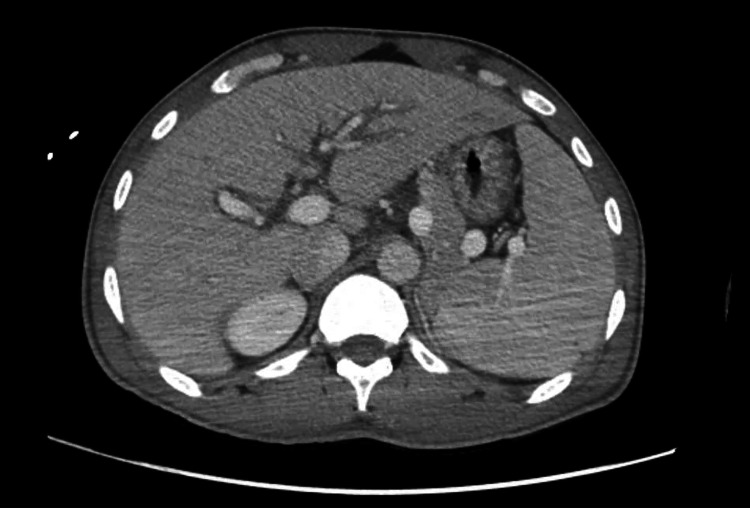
CT scan of the Abdomen and Pelvis This image depicts slight ascites, periportal edema in the liver, pericholecystic edema, hepatomegaly, and splenomegaly.
